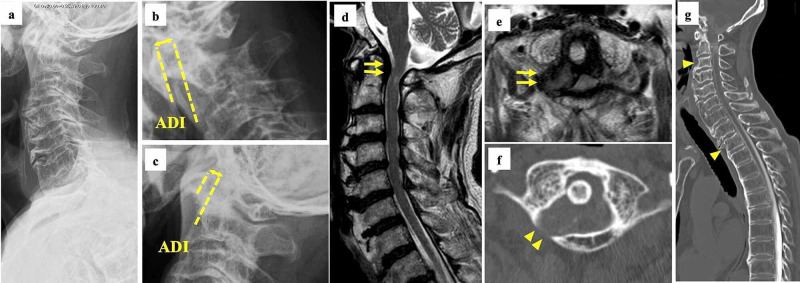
Preoperative images Neutral (a), flexion (b), and extension (c) lateral radiographs showing an increased atlas-dens interval only in the cervical flexion position, which indicates mild reducible atlantoaxial subluxation. Sagittal (d) and axial (e) MRI showing severe compression of the spinal cord at the level of C1 due to a retro-odontoid pseudotumor (arrows). Axial CT (f) revealing C1 posterior arch scalloping due to the pseudotumor (arrowheads). Sagittal CT (g) showing continuous ossification of the anterior longitudinal ligament from C3 to T2 (arrowheads) MRI: magnetic resonance imaging; CT: computed tomography
